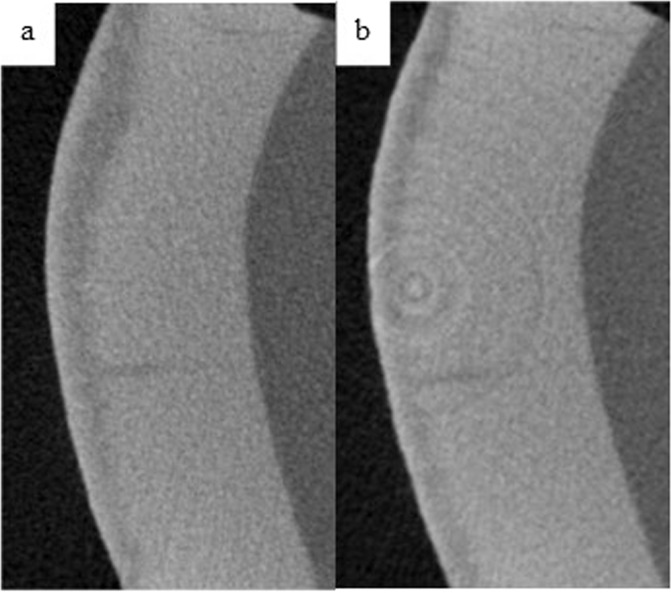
Remineralization of unetched enamel MIH lesions with 1450 ppm NaF toothpaste. Representative µ-CT images of unetched enamel MIH lesions, before (a) and after (b) in-situ remineralization.
